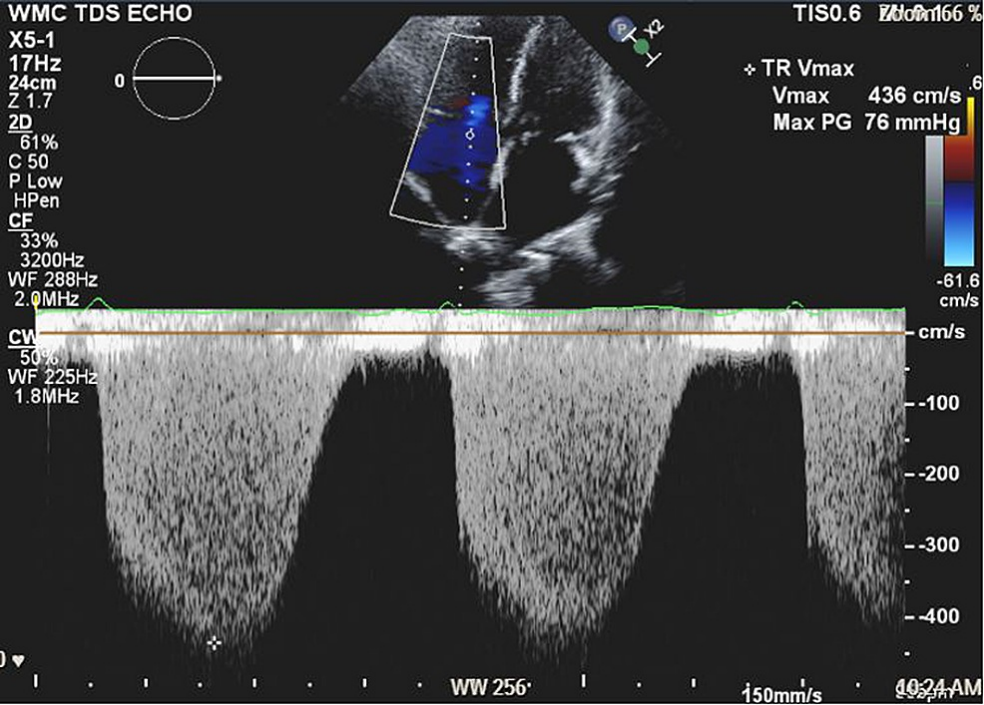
Preoperative TTE apical-chamber view with tricuspid regurgitation and dilated right ventricle. Right ventricular systolic pressure (RVSP) = 76 mmHg + right atrial pressure (RAP). TTE: transthoracic echocardiogram
